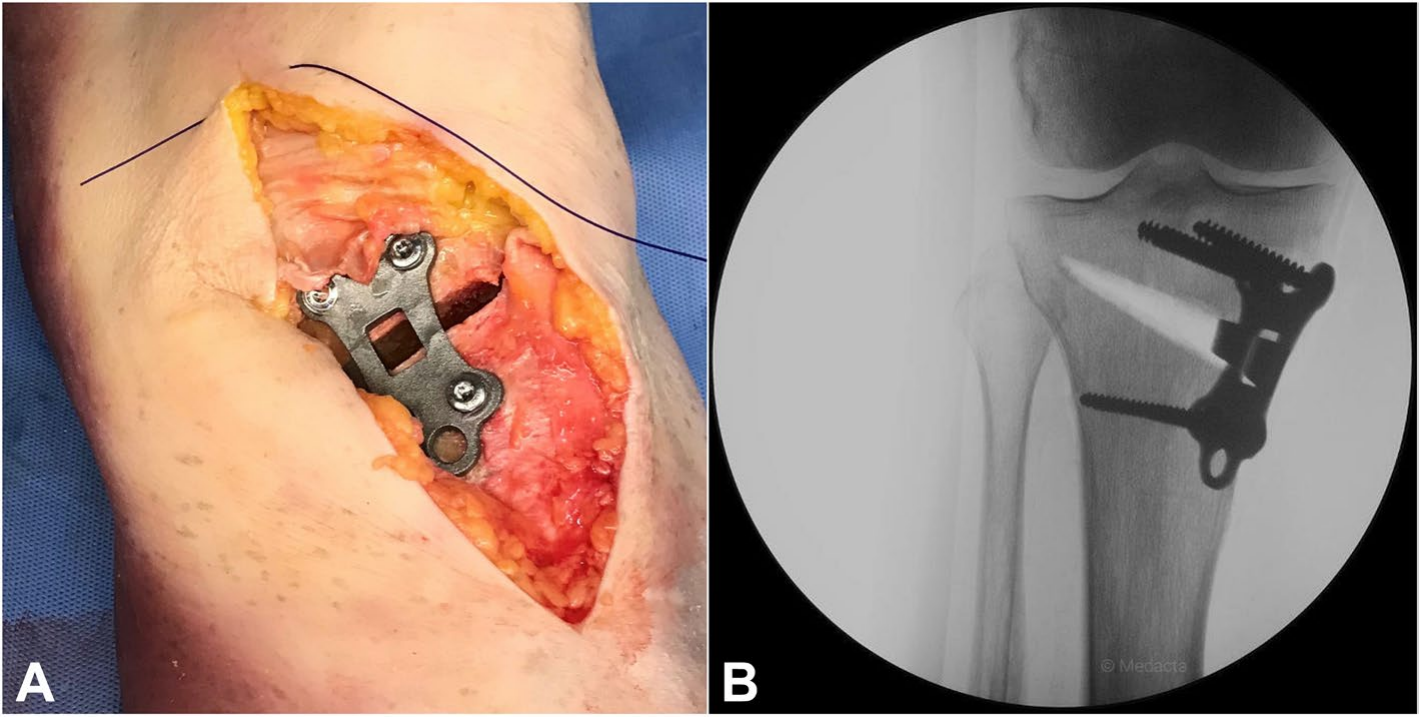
Final result. A The personalized plate in position. B Antero-posterior view of the intra-operative x-ray
